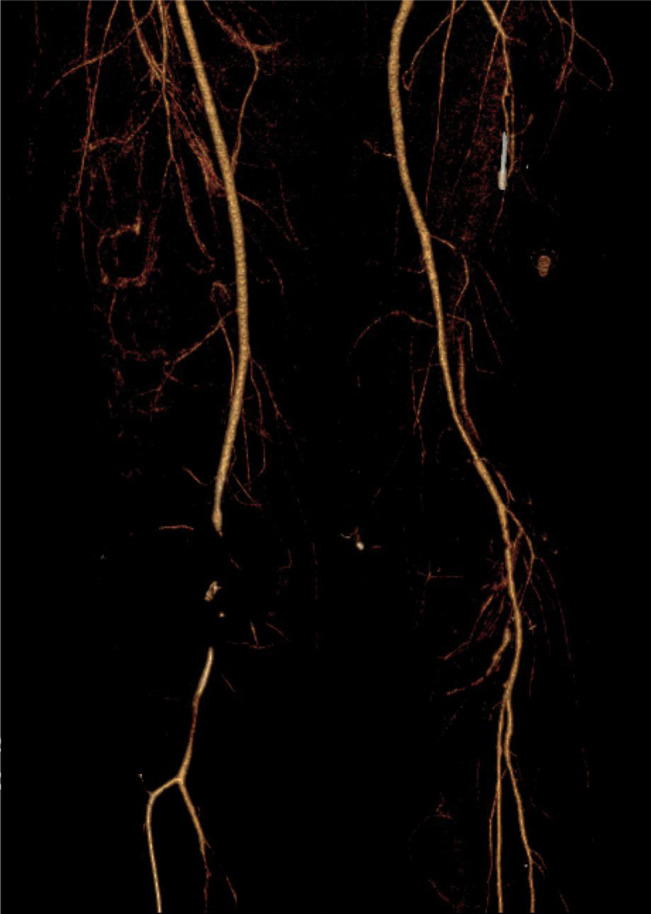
3D reconstruction of CTA demonstrating impaired runoff beginning at the proximal popliteal artery. CT image obtained at initial injury presentation.
